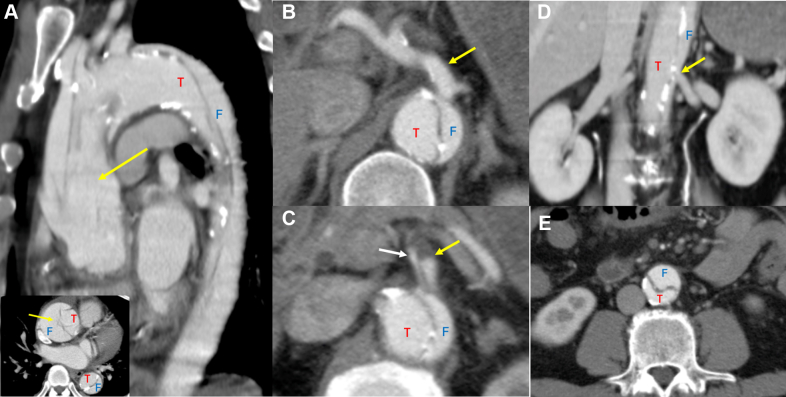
Computed Tomography Angiography at Presentation (A) Intimal tear (arrow) identified in the dissected ascending aorta with extension into the innominate artery. (B) Celiac artery perfused via the false lumen (arrow). (C) Superior mesenteric artery shows compression of the true lumen (white arrow) by a thrombosed false lumen (yellow arrow). (D) Left renal artery perfused by the true lumen (arrow). (E) True lumen compressed by the false lumen at the level of the distal abdominal aorta. F = false lumen; T = true lumen.
